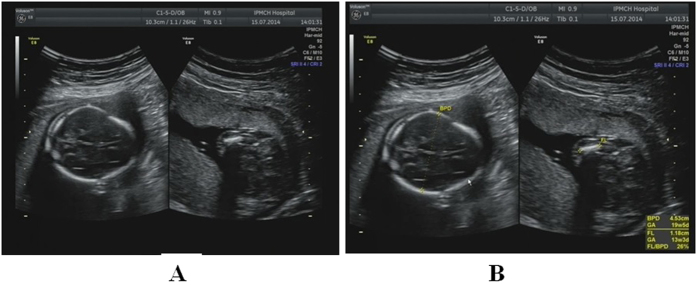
Thanatophoric dysplasia at a gestational age of 18 weeks. (A) Two-dimensional sonogram showing a biparietal diameter (BPD) of 45 mm and a thighbone length of 12 mm, indicating the shortening of all limbs. (B) The estimated gestational age of the fetus was 19 weeks and 5 days according to the BPD but 13 weeks and 3 days according to the length of the thighbone. The thighbone/BPD ratio was 26%.
